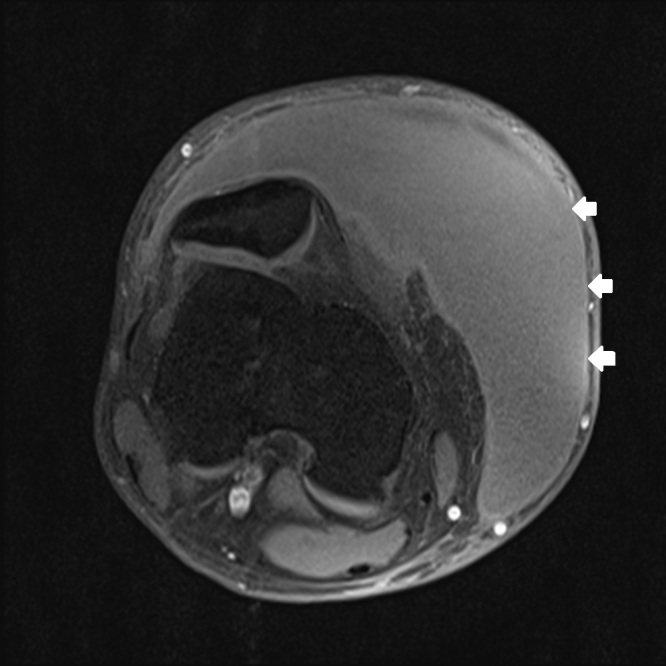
Axial T-1 weighted magnetic resonance imaging with fat saturation shows a capsule of variable thickness (white arrows).
